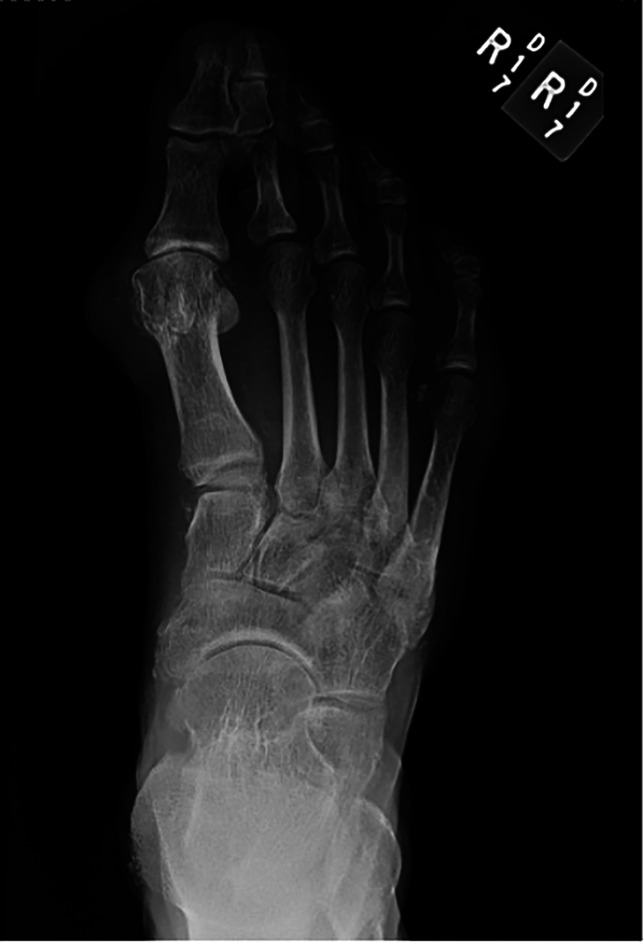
Radiographic imaging of gouty tophus associated with hallux valgus deformity showing joint destruction and medial soft tissue of tophus (author's clinical photograph).
